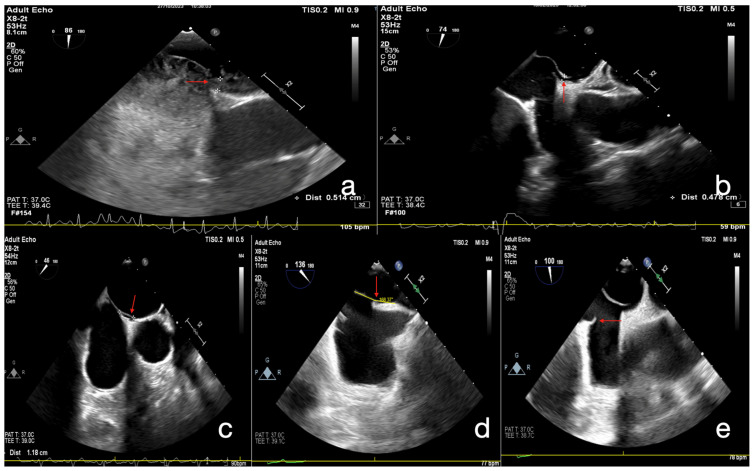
Representative echocardiographic features of high-risk PFO anatomy. (a) Contrast echocardiography demonstrating a large (>20) right-to-left bubble shunt during Valsalva maneuver. (direction indicated by an arrow). (b) Atrial septal aneurysm with marked septal excursion > 10–15 mm into either atrium (arrow). (c) PFO tunnel length, visualized as an extended overlap between septum primum and septum secundum (arrow). (d) Inferior vena cava (IVC) angle measuring 12°, with flow directed toward the interatrial septum (arrow). (e) Prominent Eustachian valve (arrow) at the junction of the IVC and right atrium, channeling blood toward the PFO.
